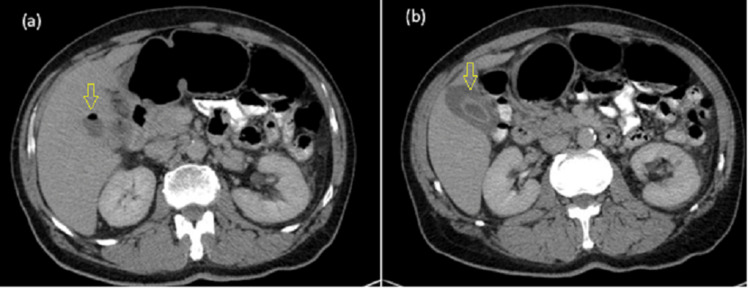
Preoperative abdominal CT with contrast shows (a) air-filled gallbladder and (b) the gallbladder revealed wall thickening and focal mural disruption, with a pericholecystic fluid collection
